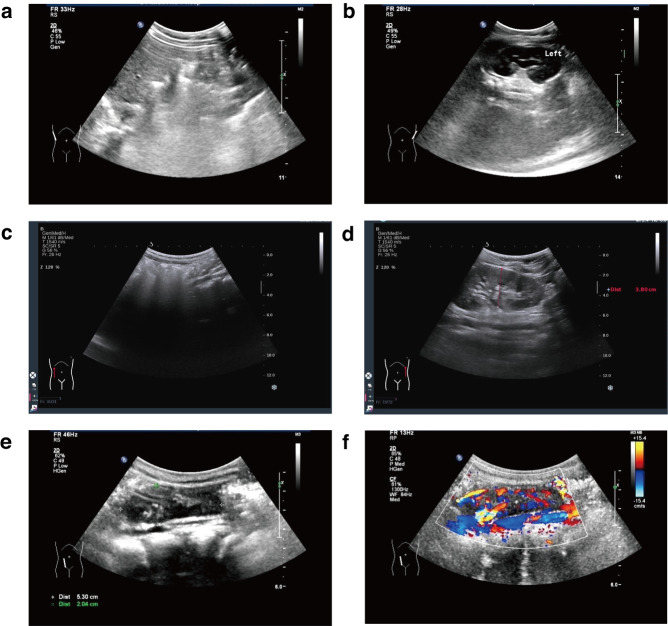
Postnatal ultrasound screening of patients URA-1, URA-6 and RE-10. a Images of patient URA-1 with a diagnosis of right renal agenesis. b By ultrasound, the left kidney of patient URA-1 can be seen, with mild hydronephrosis. c By ultrasound, patient URA-6 was diagnosed with right renal agenesis, as shown in c. d The left kidney of patient URA-6 can be seen in d. e Patient RE-10 with a diagnosis of right renal ectopy. On ultrasound, right renal echoes were seen in the right iliac fossa, as shown in e. f The blood flow signal of the right kidney of patient RE-10 was normal, as indicated in f.
